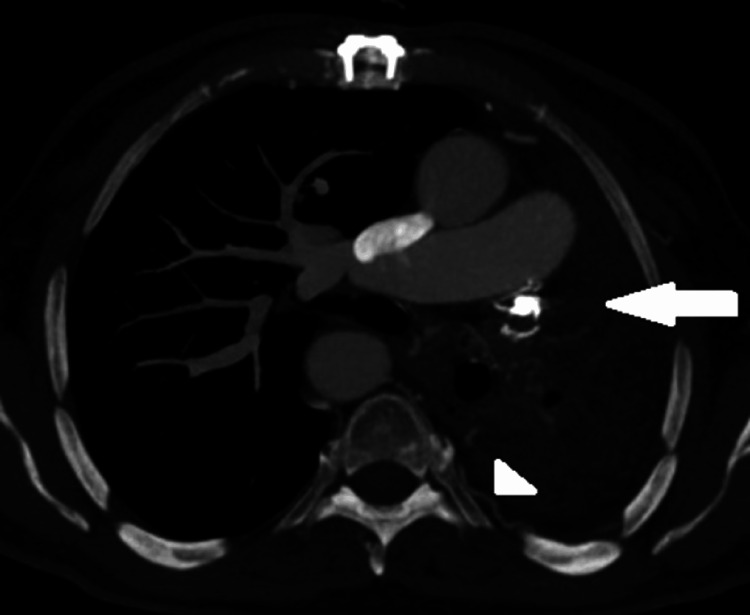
Axial soft tissue window. The CT angiographic image of the chest demonstrates unilateral absence of the left pulmonary artery (white arrow). Numerous tiny hypertrophied bronchial collateral vessels are seen within the left lung (arrowhead).
